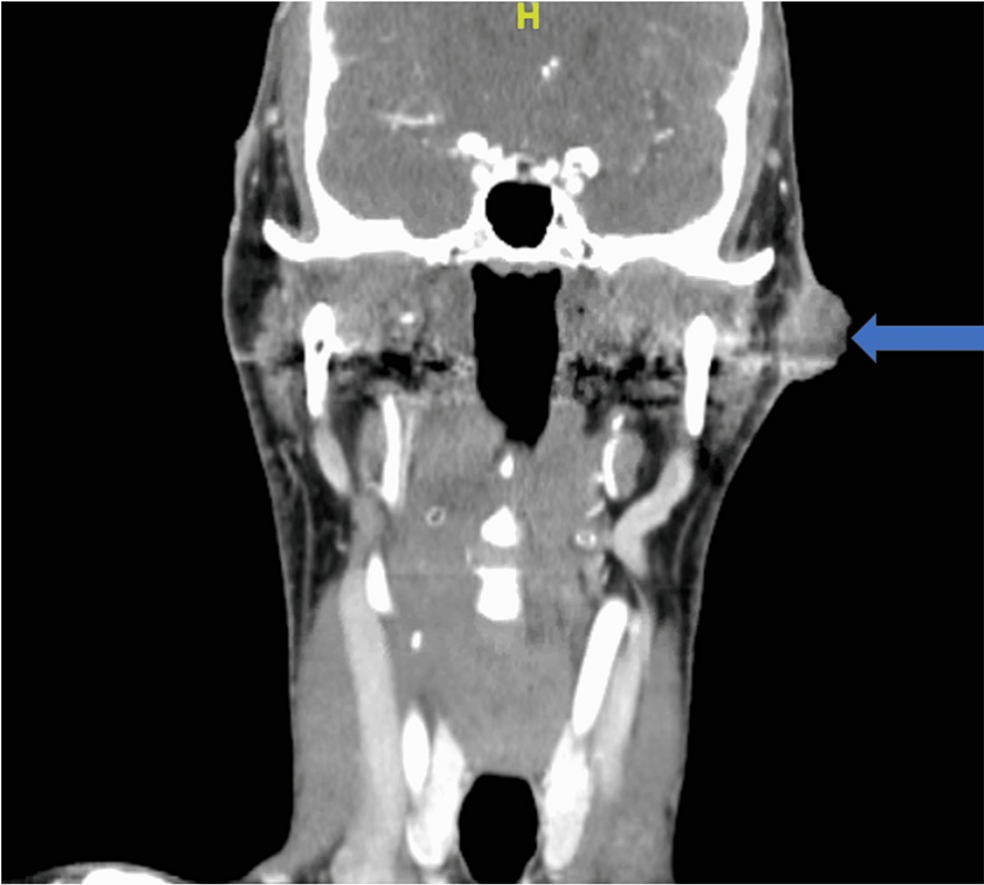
CT of the left neck (coronal view) demonstrates 3.2 x 1.9 x 2.5 cm fungating mass with concerns for malignancy (blue arrow)
